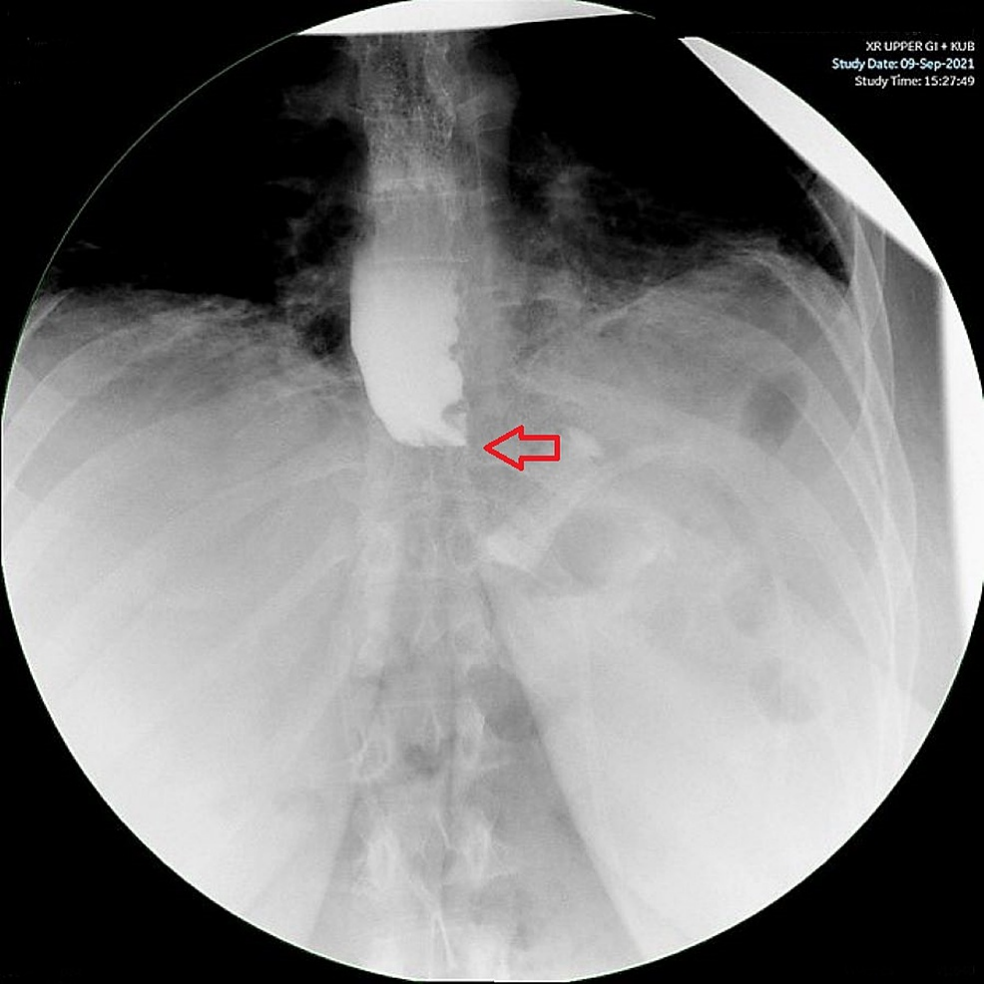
Upper GI radiograph showing retention of contrast, indicating proper function of gastric band and no leakage (red arrow)
